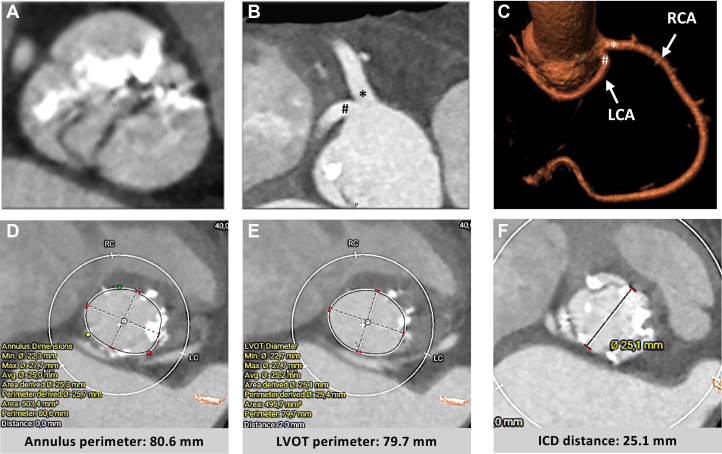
Preprocedural Computed Tomography Analysis (A) Calcified bicuspid aortic valve Sievers type 1 with raphe between the RCC and NCC. (B) Aberrant origin of the left coronary artery (#) originating from the RCC. (C) Three-dimensional CT reconstruction demonstrating a retroaortic course of the left coronary artery. (D) Aortic annulus dimensions. (E) Left ventricular outflow tract dimensions. (F) The intercommissural distance as measured 4 mm above the annulus. CT = computed tomography; ICD = intercommissural distance; LCA = left coronary artery; LVOT = left ventricular outflow tract; NCC = noncoronary cusp; RCA = right coronary artery; RCC = right coronary cusp.
